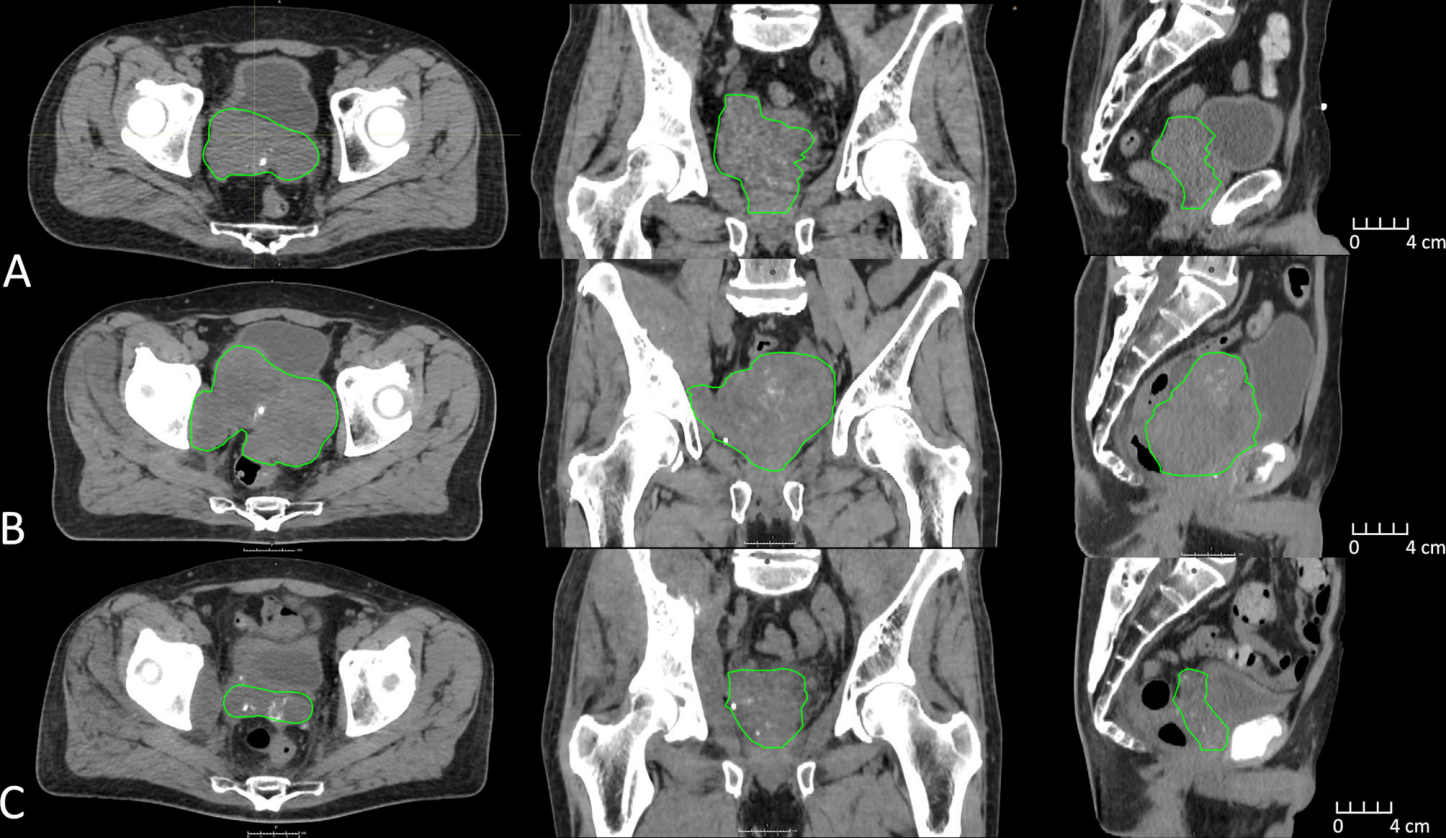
CT imaging depicting clinical target volume (green) changes approximately 1 year prior to MR-Linac treatment (A), immediately prior to MR-Linac treatment (B), and 6 months post MR-Linac treatment (C).
